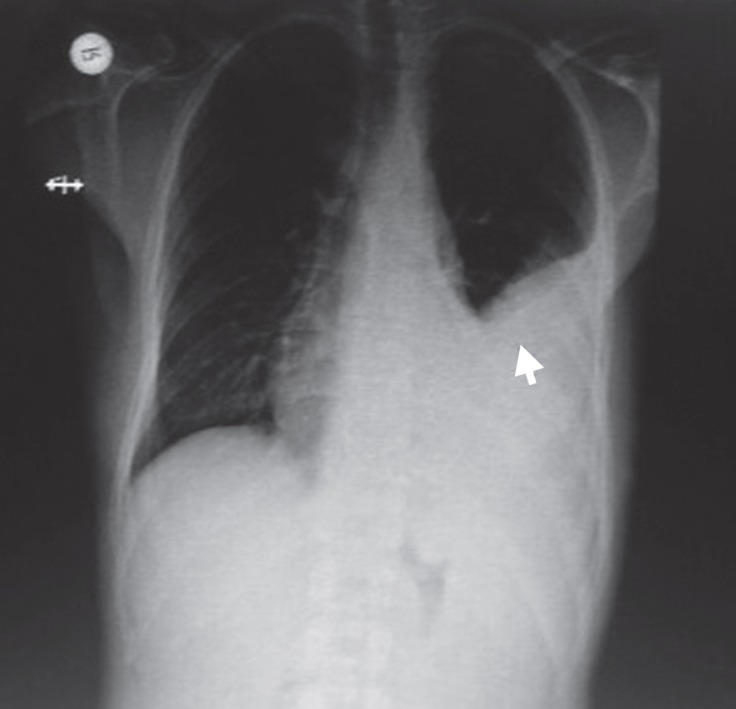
Posteroanterior chest radiography, on admission, shows a consolidated region in the left thoracic cavity (arrow).
